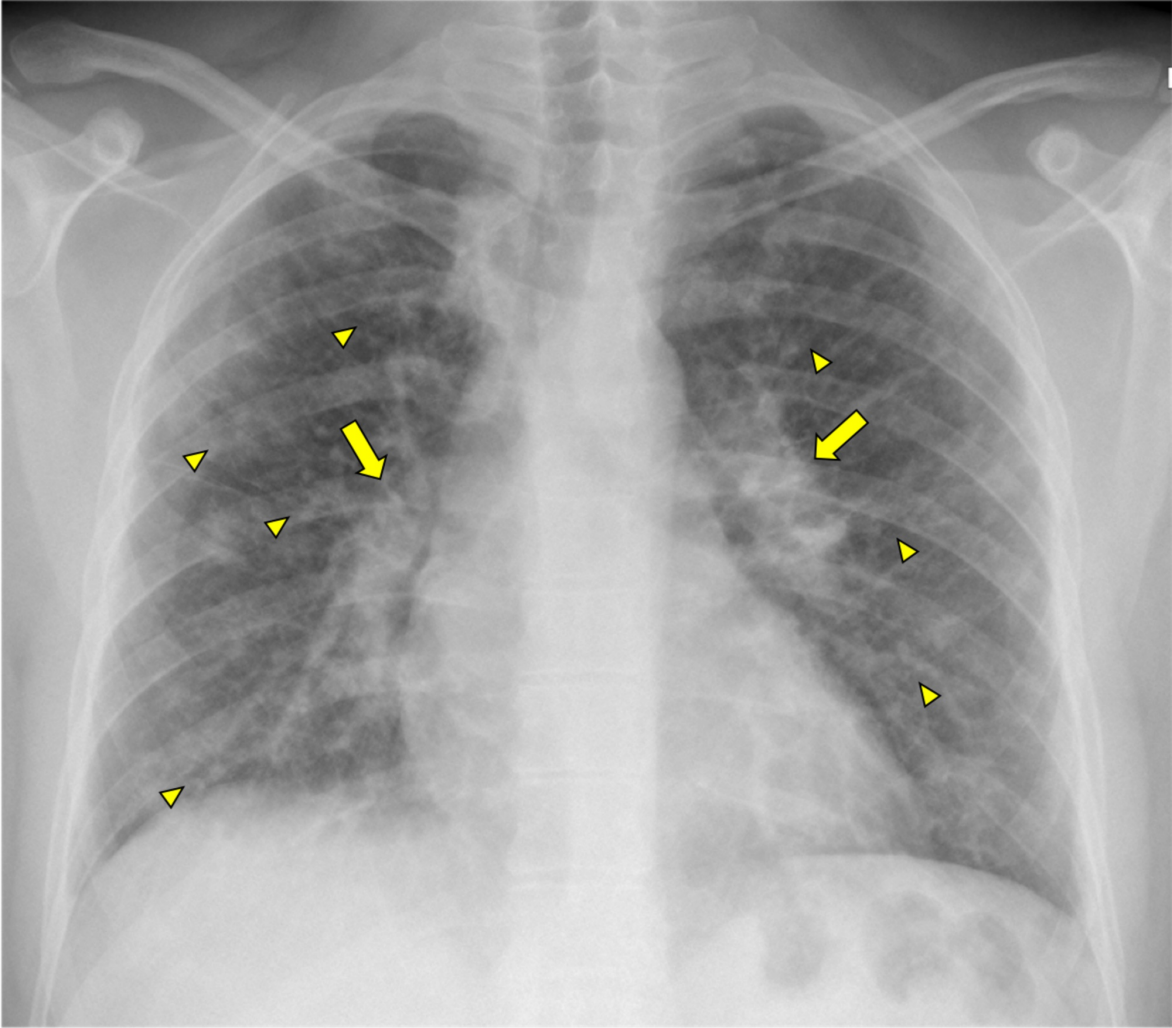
Chest X-ray: bilateral hilar enlargement (arrows) and reticulonodular opacities (arrowheads)
